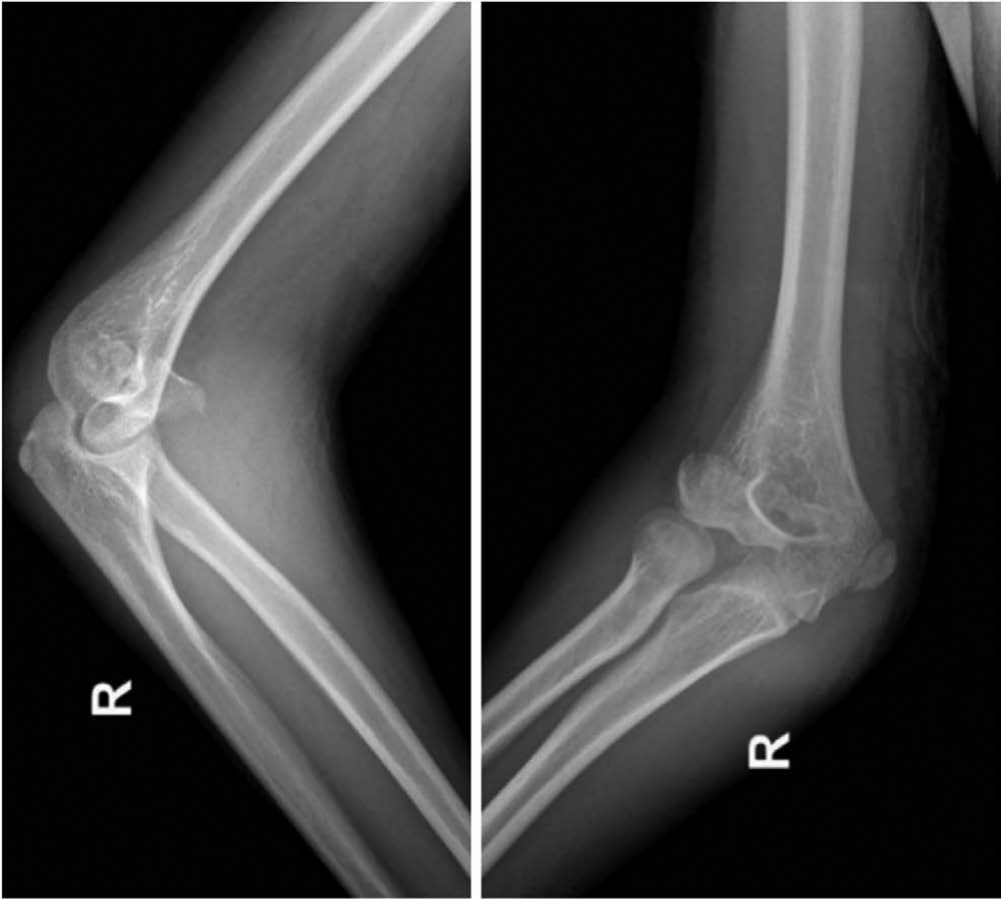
X-ray of the right elbow.
